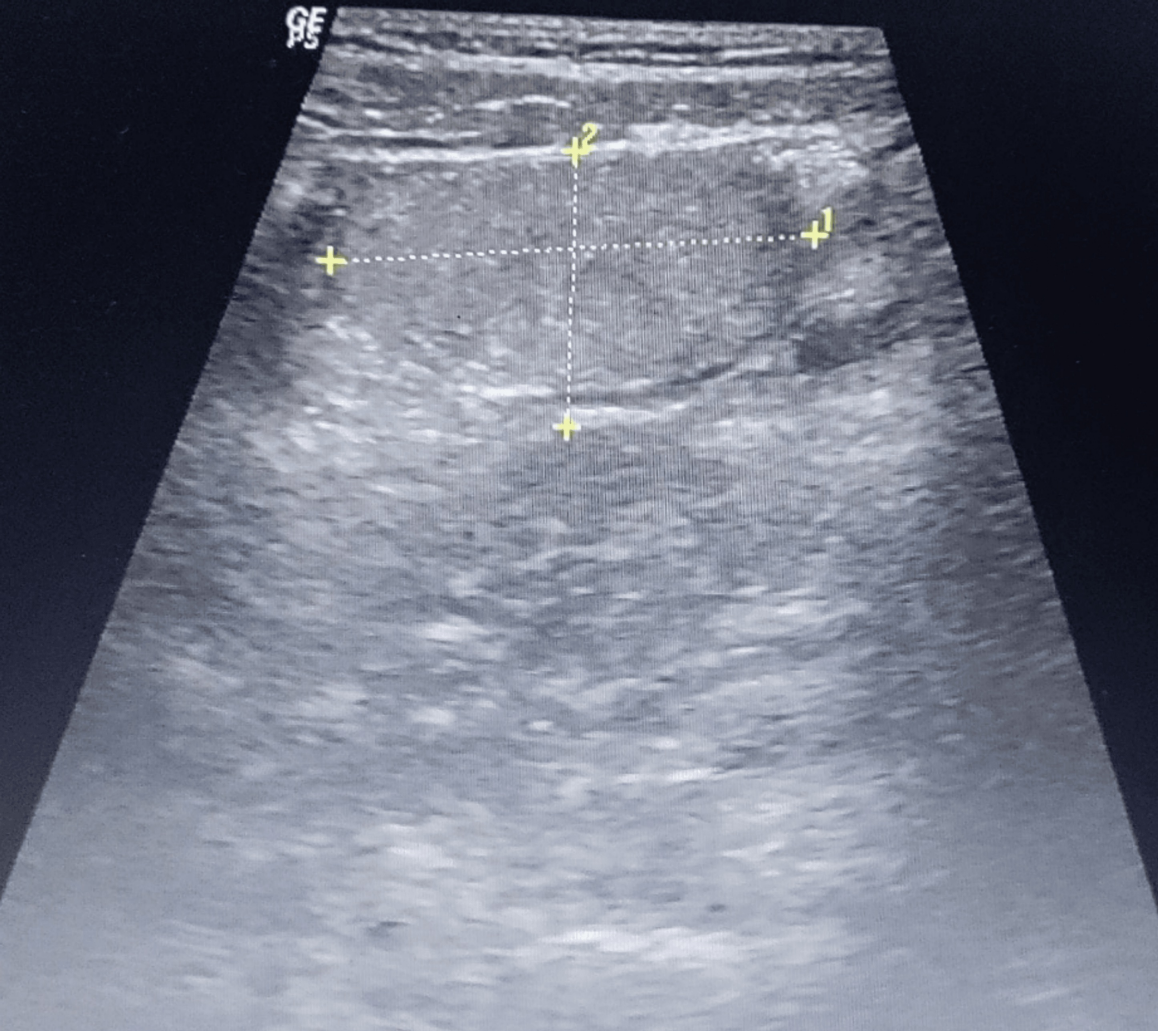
Ultrasound showing an isoechoic area in the right inguinal fossa
